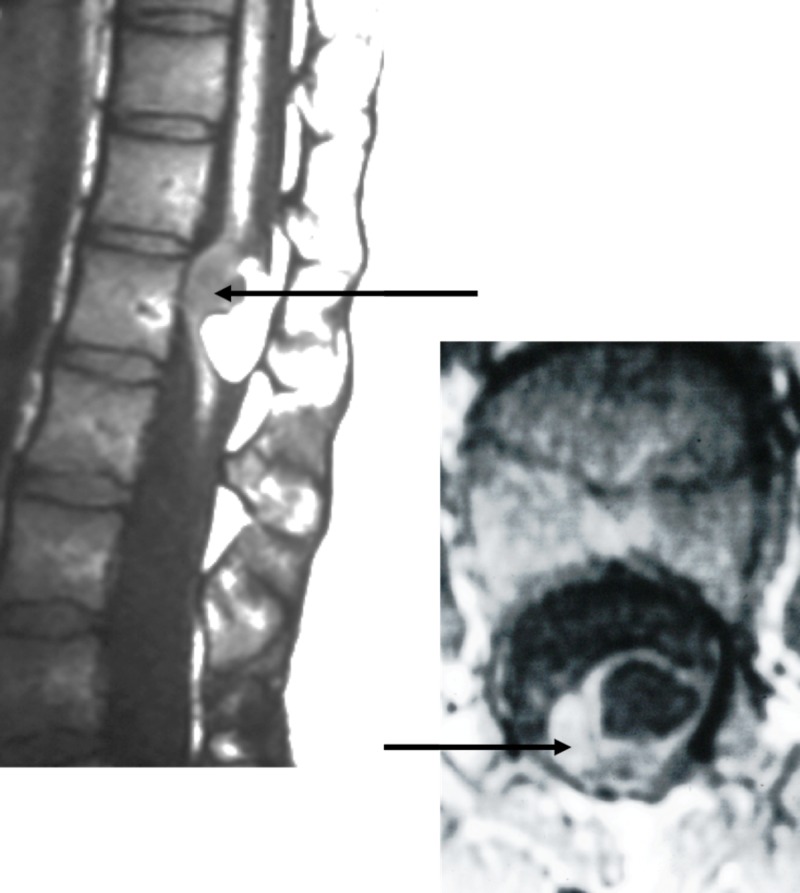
Left: Sagittal T1-weighted magnetic resonance imaging of the patient found to harbor a spinal neurenteric cyst (NEC) (arrow). Also note the dorsal lipoma posterior to the NEC; Right: Axial T2-weighted MRI of this patient depicting the NEC (arrow).
